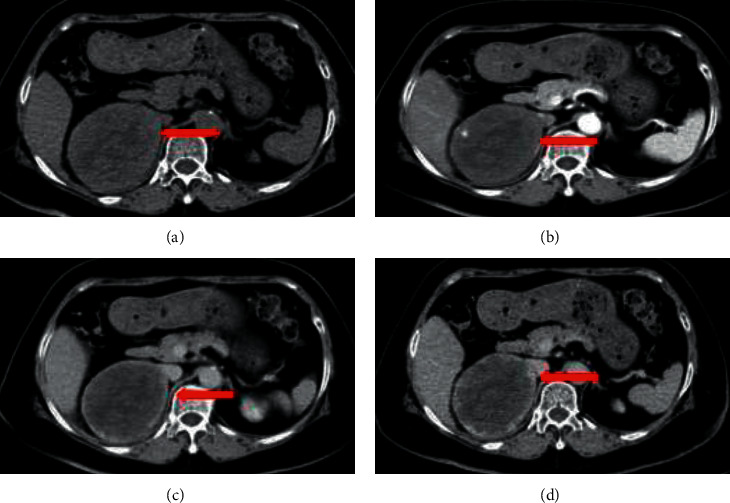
Right adrenal cavernous hemangioma with a tumor size of 10 cm. CT showing mild heterogeneous enhancement of the tumor during the arterial phase and progressive enhancement in the venous phase and the delayed phase (red arrow). (a) Enhanced CT. (b) Arterial phase of CT. (c) Venous phase of CT. (d) Delayed phase of CT.
